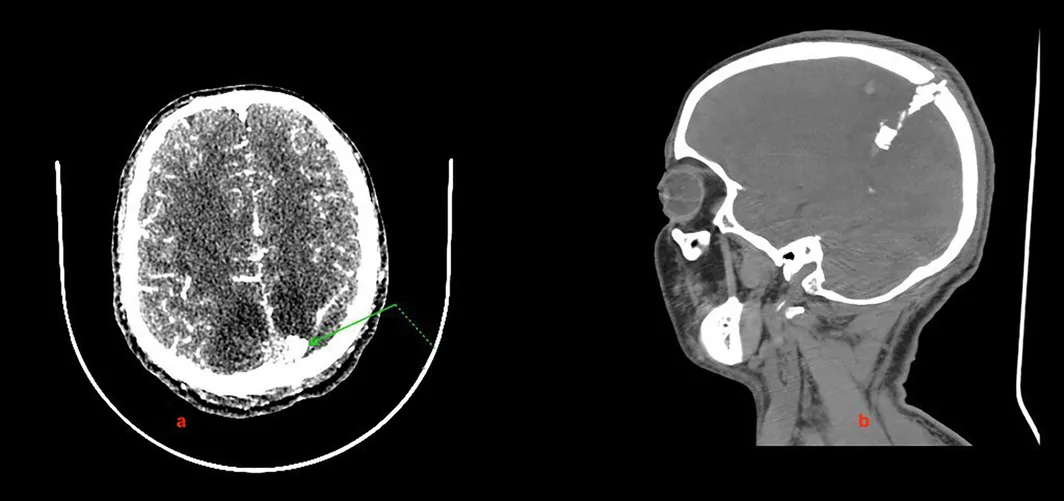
Contrast‐Enhanced Brain CT Venography. Axial (a) and sagittal (b) images from a CT venogram. The axial image represents contrast flow around a central thrombus. The sagittal image (b) confirms a long‐segment filling defect within the superior sagittal sinus extending to the confluence of sinuses.
